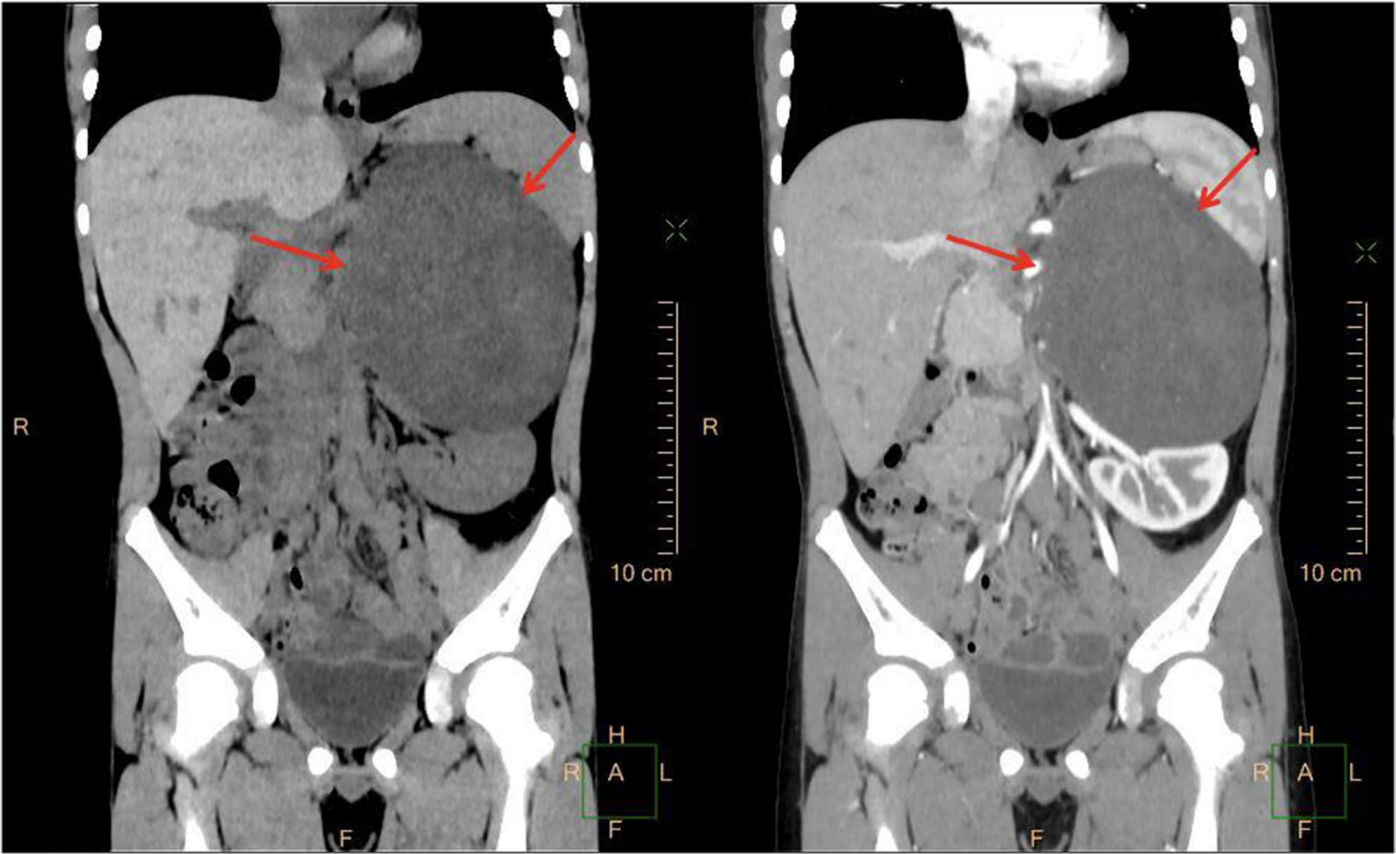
CT showed a large oval mass of homogenous soft-tissue density above the left kidney with slight enhancement
